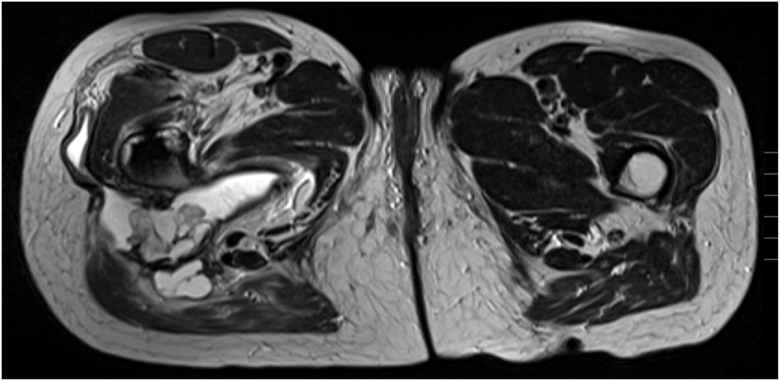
Axial view of an MRI with T2 weighting and TSE sequencing in December 2023 at the level of the pseudocyst in the right thigh, in direct contact with the sciatic nerve. TSE, turbo spin echo.
